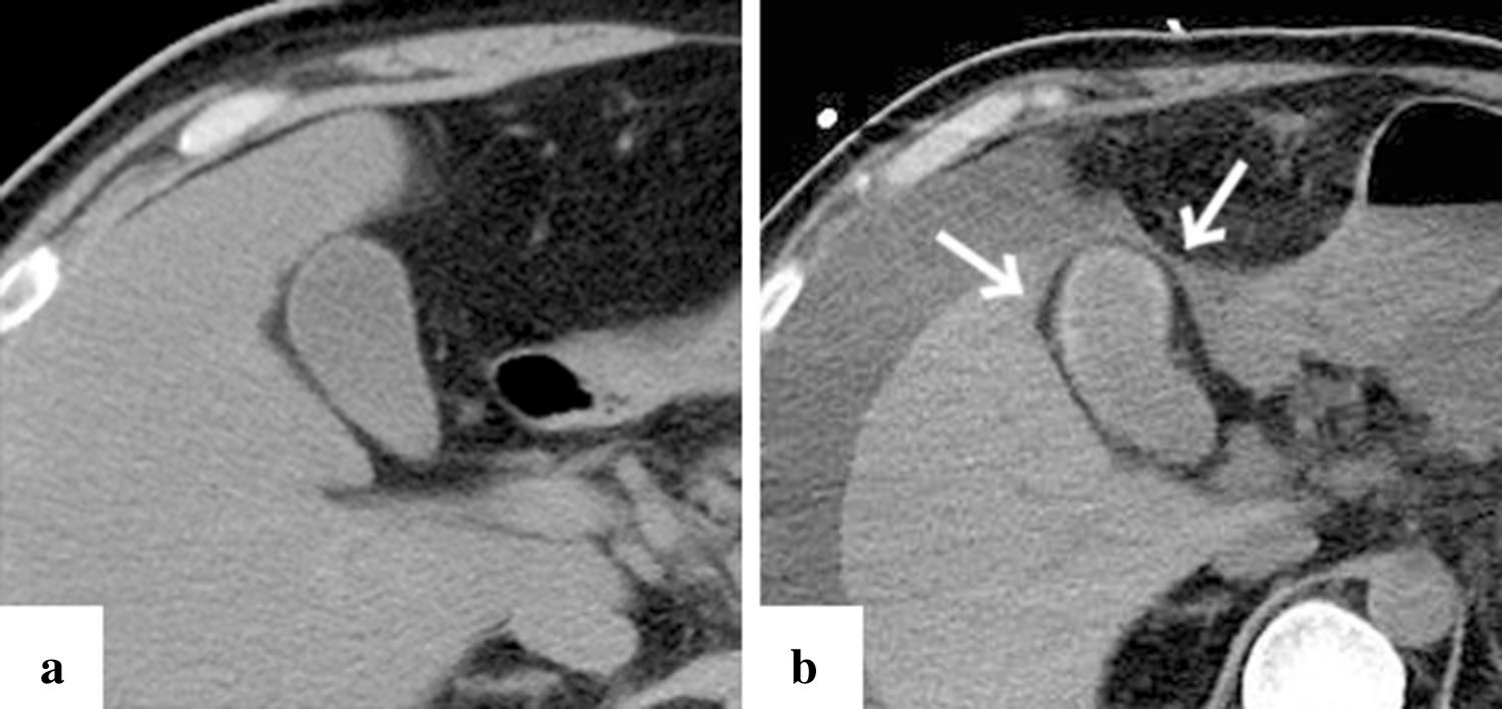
Representative case of gallbladder calcification in a 51-year-old patient (case no. 13). Computed tomography (CT) imaging on day 1 since the onset of symptoms (a) and after one month of the admission (b). One month after admission, thickening of the gallbladder wall was not observed and calcification of the wall occurred mainly at the base of the gallbladder (b, white arrow)
